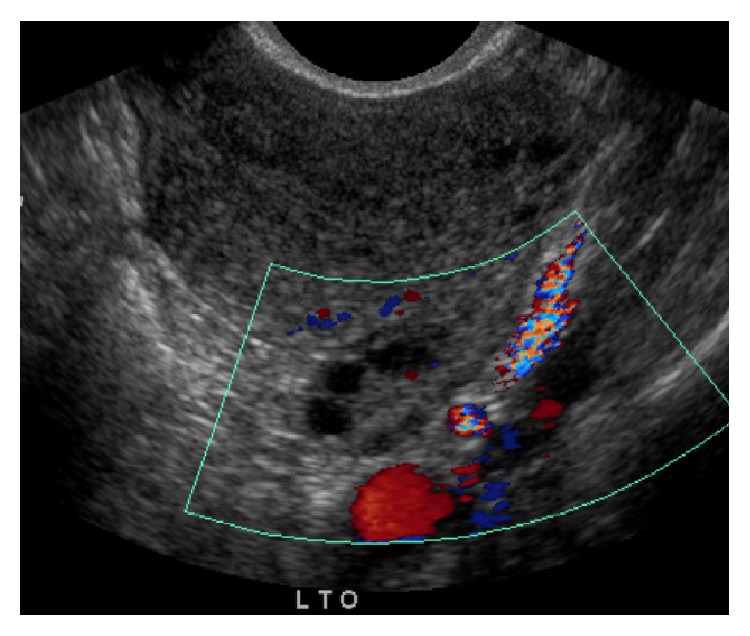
Pelvic ultrasound 5/31/13: unremarkable scan, picture showing normal left ovary with the normal Doppler flow.
